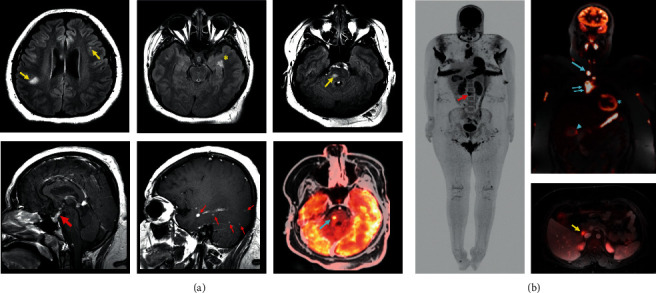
Disease status prior to intervention with 2CdA. (a) Brain MRI: multiple focal lesions are seen at the subcortical white matter and brainstem (yellow arrows), cerebellum (not shown), and left mesial temporal lobe/amygdala (^*∗*^) on T2 FLAIR imaging. Enhancing soft tissue is also seen at the pituitary stalk extending into the sella (big red arrow). Additional enhancing nodules (small red arrows) are seen in the brain. Corresponding abnormal FDG uptake (teal arrow) is also demonstrated from a brain image in the whole-body PET-MRI. (b) Whole-body PET MRI: multiple foci of abnormal FDG activity are seen in the neck, including the right thyroid (long teal arrow) and in the mediastinum along the thymic tissues (double teal arrows). Diffuse mucosal thickening of the gastric wall (^*∗*^) and gallbladder wall (arrowhead) with FDG-avidity also suggested possible XD involvement and/or inflammation. Enlarged and FDG avid portocaval lymph node (yellow arrow) and involvement of the vertebral body (red arrow) were also present.
